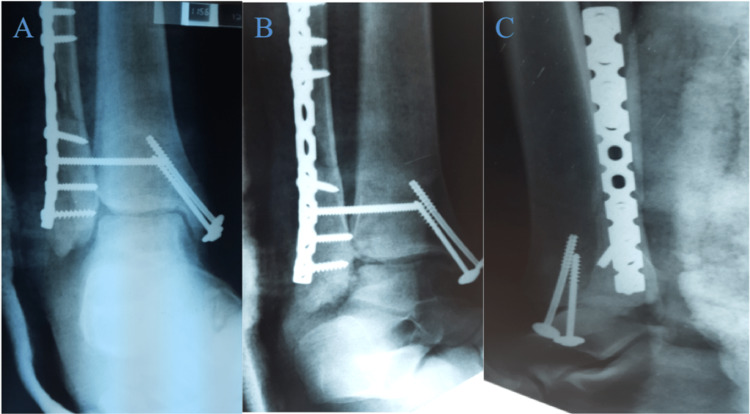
Post-operative radiographs (AP, mortise and lateral views) A - AP view, B - mortise view, C - lateral view AP: anteroposterior
